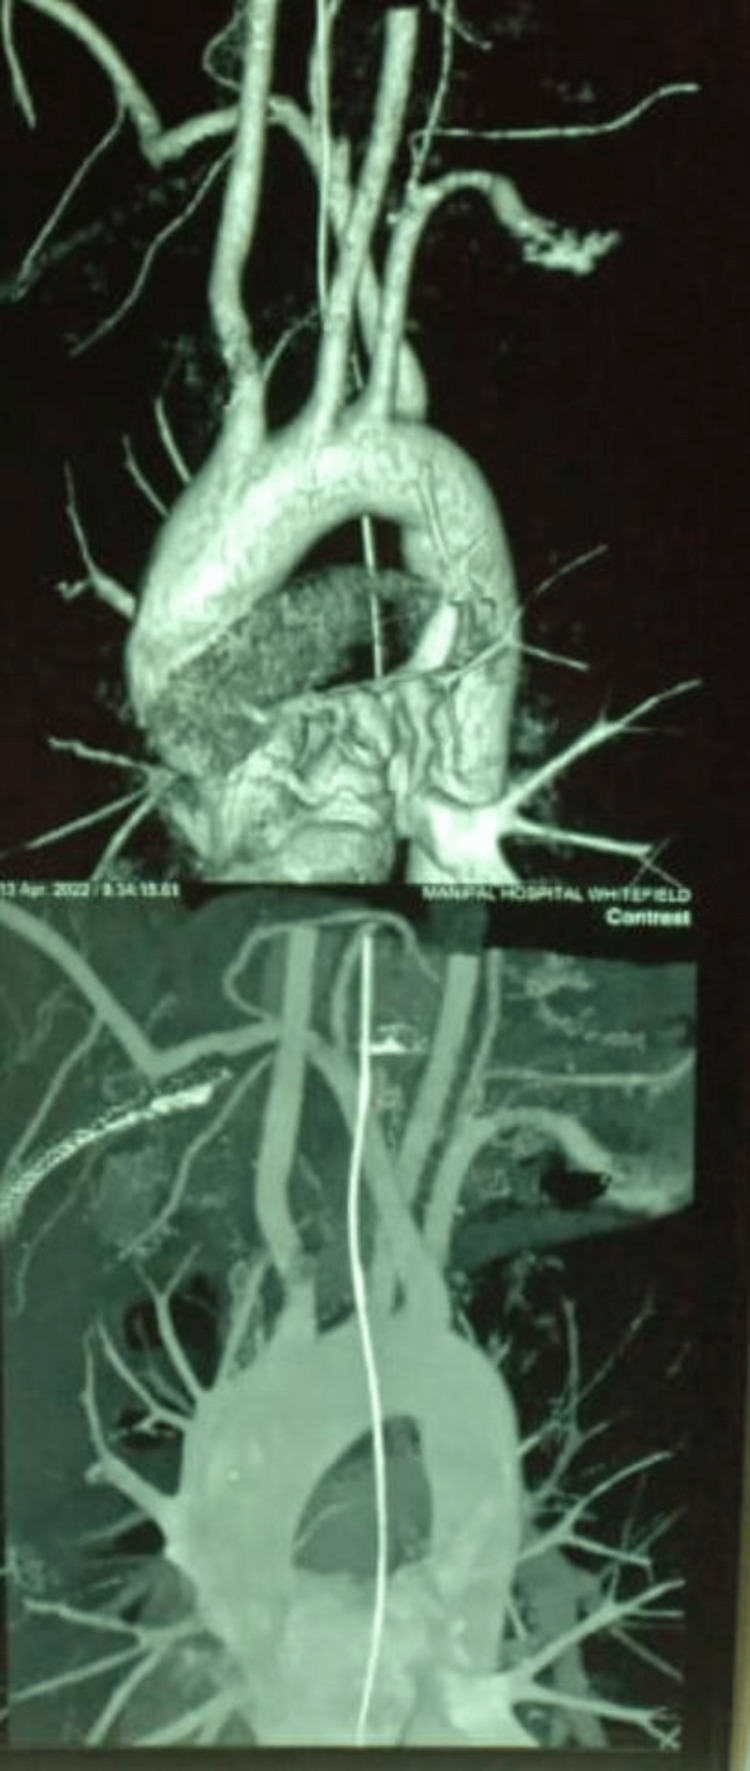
CT angiogram of the chest showing aberrant right subclavian artery
